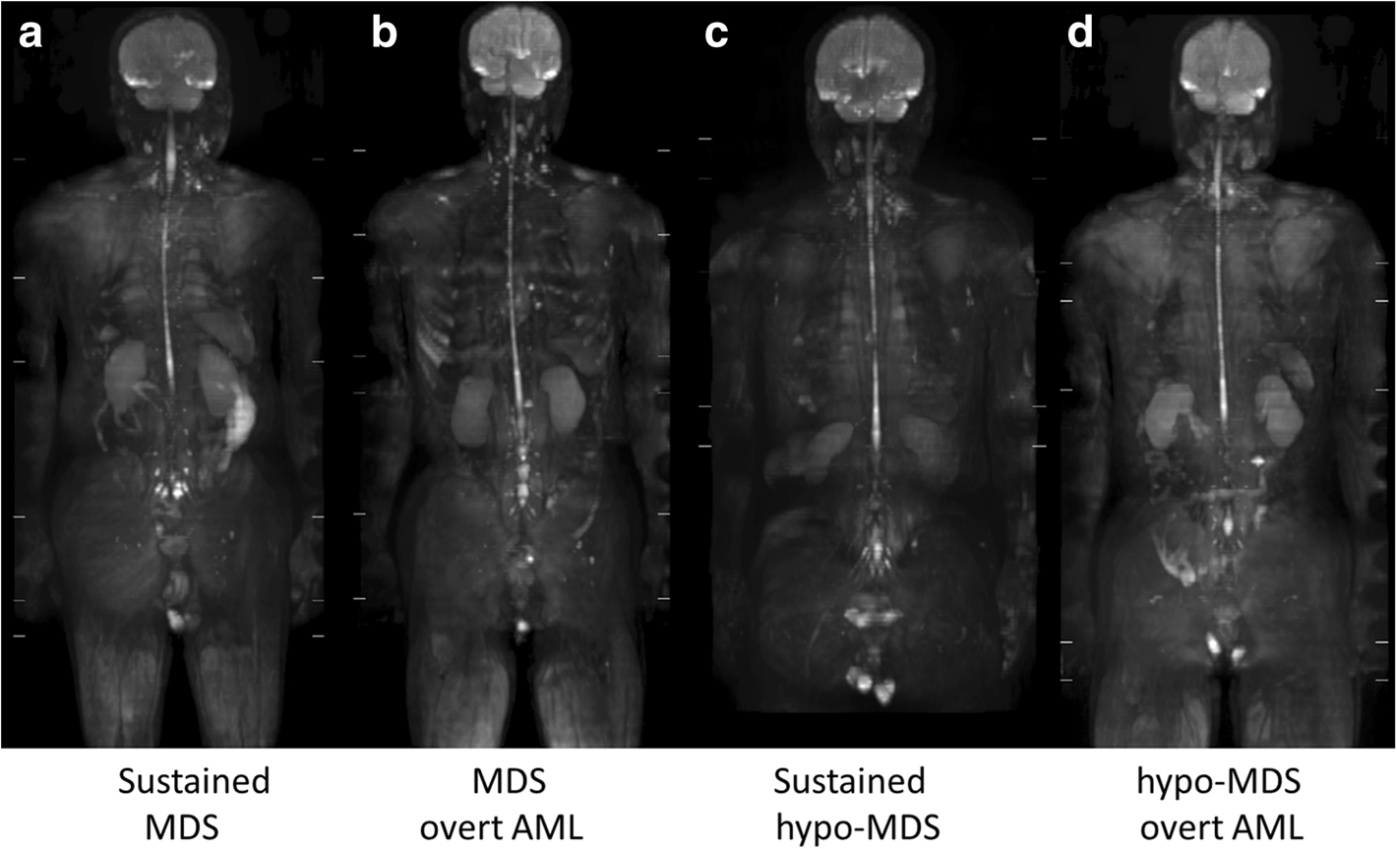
Maximum intensity projection DWI images of MDS patients (a–d). a–d in Fig. 7 are identical to a–d in Fig. 5, respectively. Whole-body DWI did not demonstrate any significant differences in bone marrow signals between MDS and hypo-MDS patients. DWI did not demonstrate any significant differences between patients with sustained MDS or overt AML
